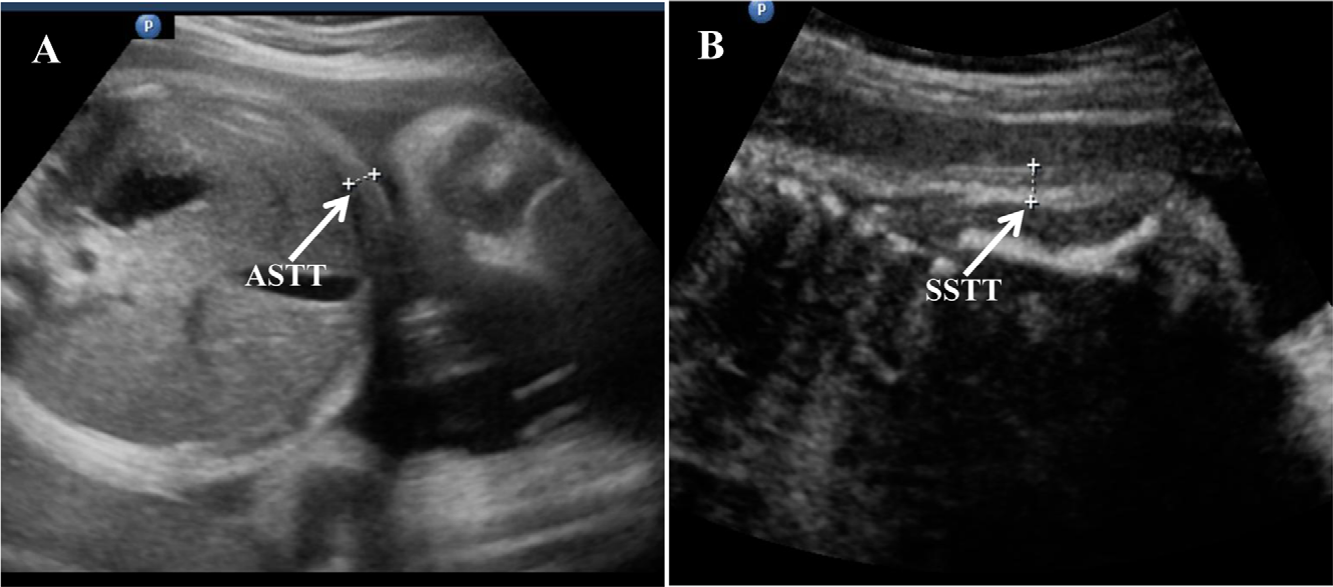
Ultrasound images of ASTT and SSTT. Images with arrows showing the measurement of (A) fetal abdominal subcutaneous tissue thickness (ASTT) and (B) fetal subscapular subcutaneous tissue thickness (SSTT).
